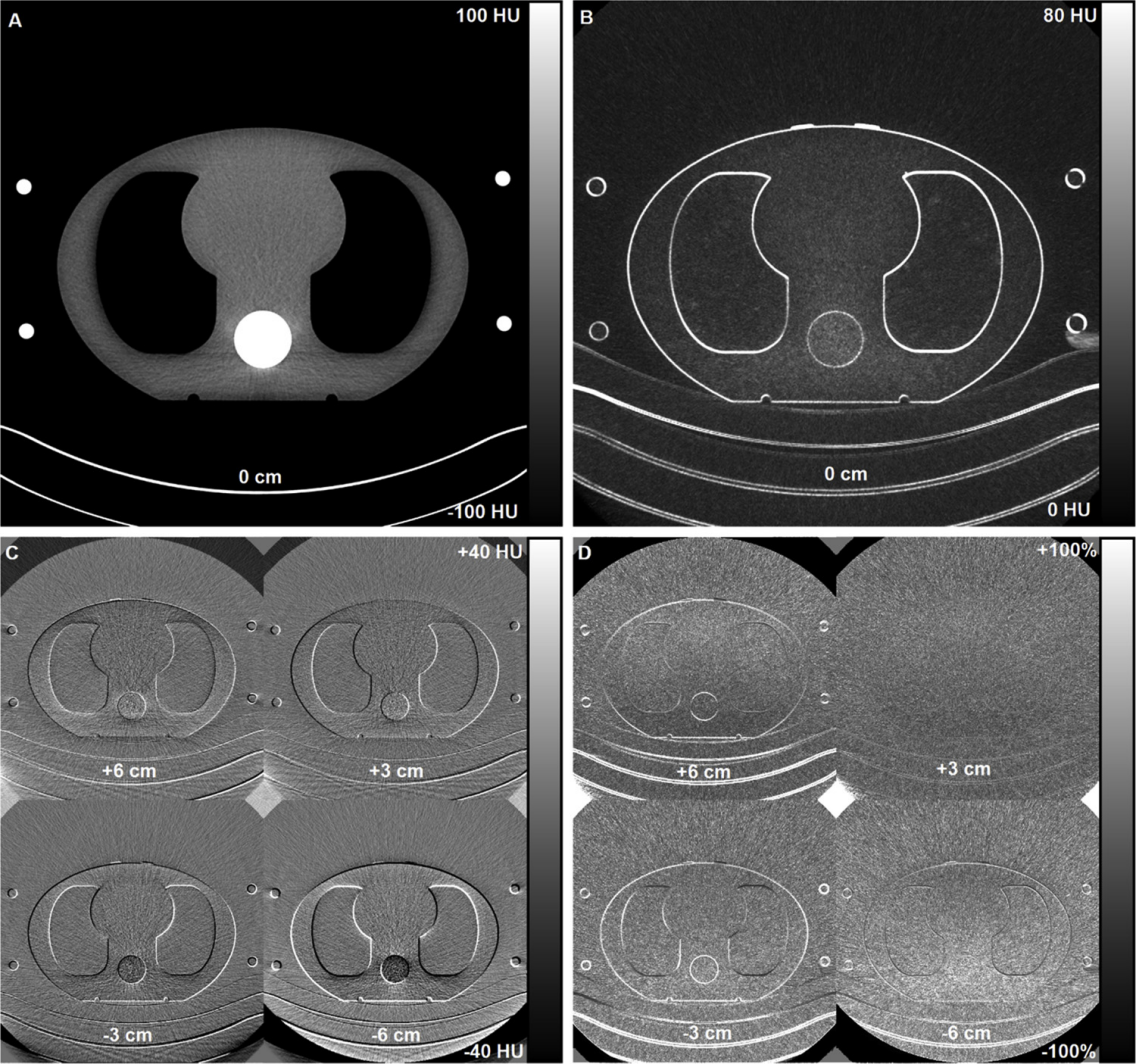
Axial image data from the Siemens chest CT scan with a fixed 120 kVp tube voltage after an AP scout. The reference axial CT image with mean CT numbers (A) and corresponding 1SD noise map (B) were calculated from ten slices comprising a total z-direction volume coverage of 10 cm. Absolute CT number differences (C) and relative noise difference maps (D) at table heights +6, +3, -3, and -6 cm were calculated relative to the reference centering (0 cm).
